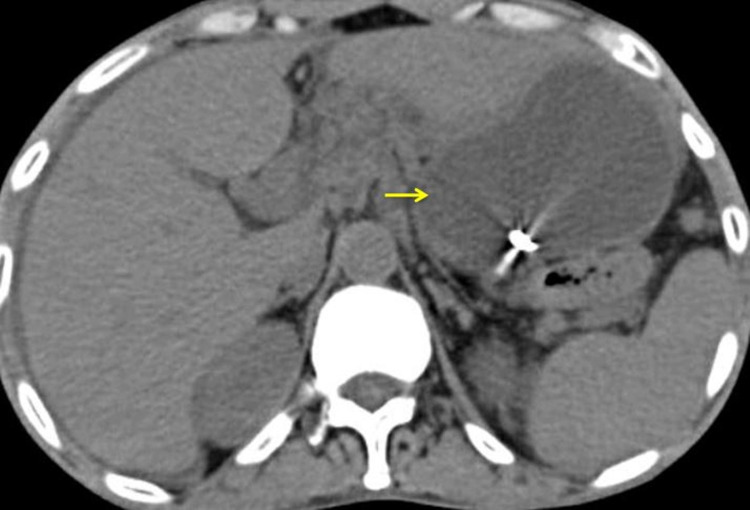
Computed tomography (axial view) done after the embolization showing coil in the left gastric artery.
